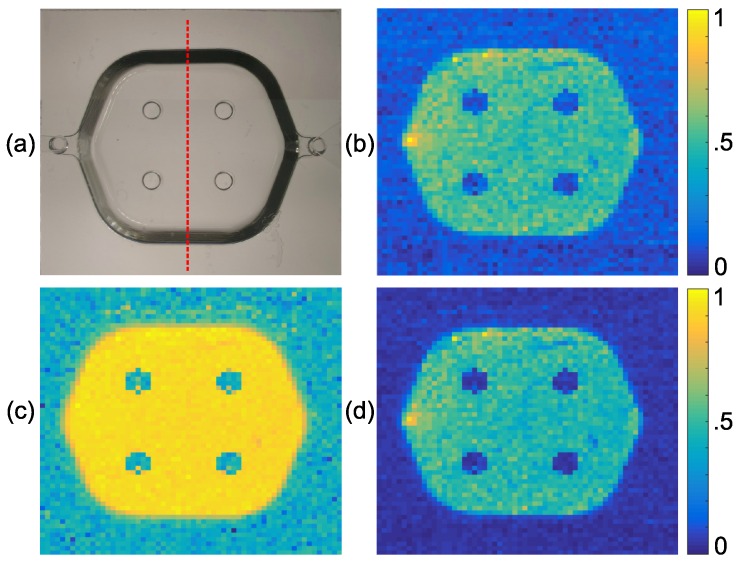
(a) Top view of the microfluidic channel (the red broken line shows the center-crossing axis alone which SBR was calculated) and experimental normalized (b) Doppler flowmetry, (c) confocal reflectance and (d) multiplication images.
